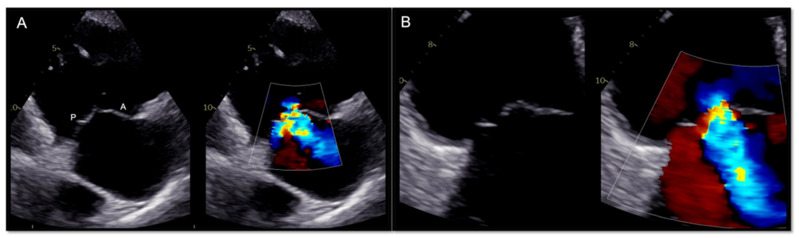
Transthoracic echocardiogram in two dimensions. Parasternal long axis RV-inflow view (A,B). Since the interventricular septum and the coronary sinus ostium are not visualized, the leaflets imaged are the posterior (P) and anterior (A); Color doppler documented severe functional tricuspid regurgitation (TR).
